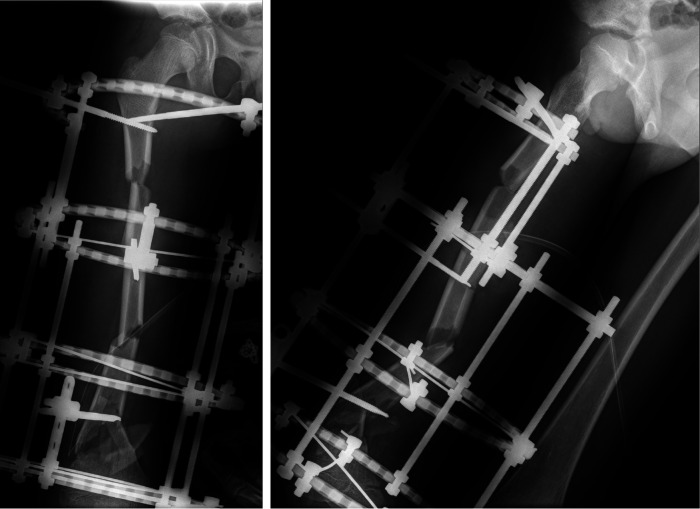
On the 5th postoperative day, shows a right lateral view x-ray of the femur, indicating a generally restored femoral alignment, but still presenting some degree of angular deformity.
